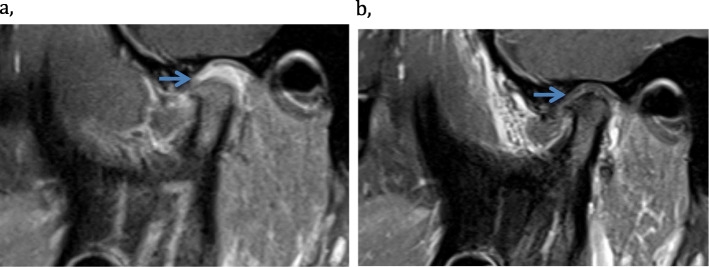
MRI improvement of the inflammation. Oblique sagittal contrast enhanced T1 TSE images with fat suppression of a 16-year-old girl (case 8) (a) at baseline with increased temporomandibular joint enhancement (blue arrow) and (b) at 2 months follow-up after IAC and no DMARDs with complete regression of joint enhancement (blue arrow). Note also the disrupted disc and flattened condyle in both images
